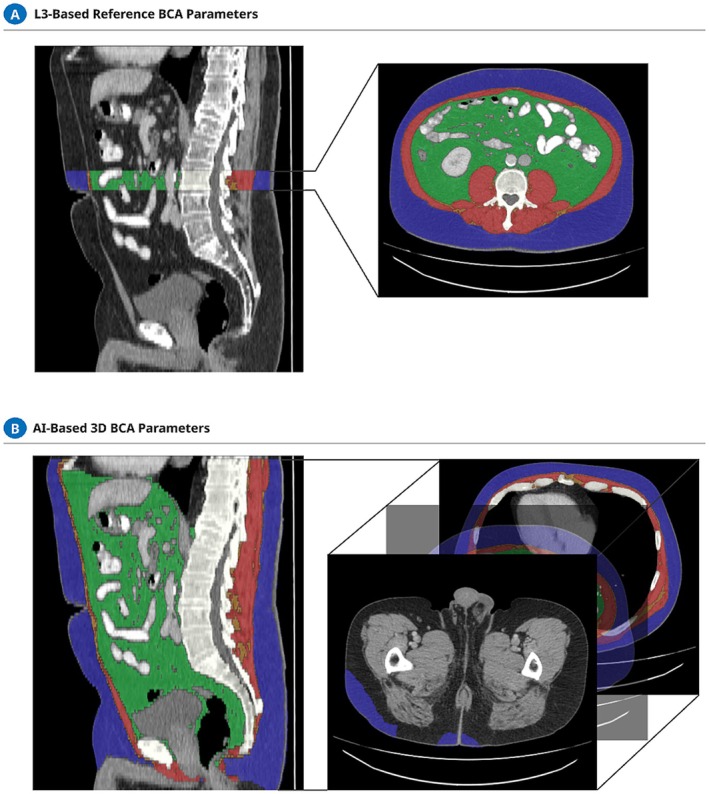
Comparison of conventional L3‐based and AI‐based volumetric body composition analysis. (a) L3‐based reference approach: sagittal CT reconstruction (left) showing the L3 vertebral level used for single‐slice tissue quantification, with corresponding axial segmentation at L3 (right). (b) AI‐based volumetric approach: sagittal view (left) and axial stack (right) demonstrating automated segmentation across the entire abdominal cavity. Tissue compartments are colour‐coded: muscle (red), subcutaneous adipose tissue (blue), visceral adipose tissue (green), intramuscular adipose tissue (orange) and bone (white). The volumetric approach captures total tissue volumes rather than cross‐sectional areas at a single anatomical landmark, providing a more comprehensive assessment of body composition.
